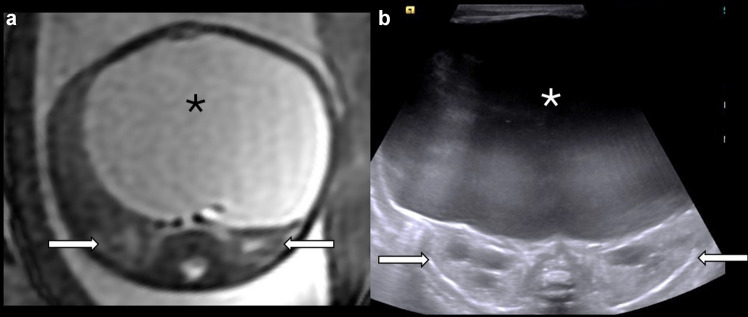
Prenatal and postnatal imaging of the bladder and kidneys in a female patient diagnosed with MMIHS. (a) An axial T2-weighted image through the fetal abdomen obtained at 25 weeks 6 days shows megacystis (*asterisk*) and mild upper urinary tract dilation bilaterally (*arrows*). (b) A transverse renal US image performed in the same patient on day of life 0 shows massive bladder enlargement (*asterisk*) and mild upper urinary tract dilation bilaterally (*arrows*)
